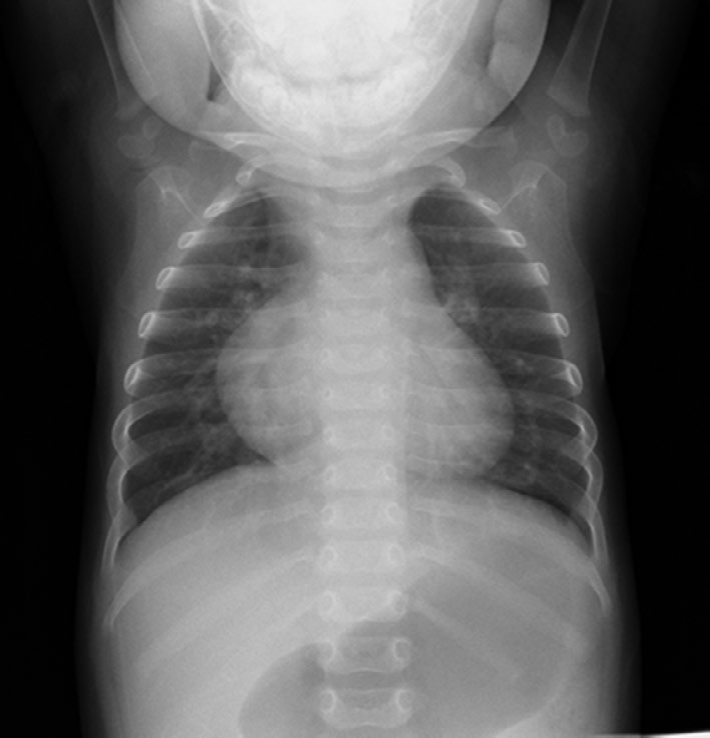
Preoperative chest radiograph demonstrating cardiac enlargement with enlargement of the right second arch and the left second‐to‐third arch
